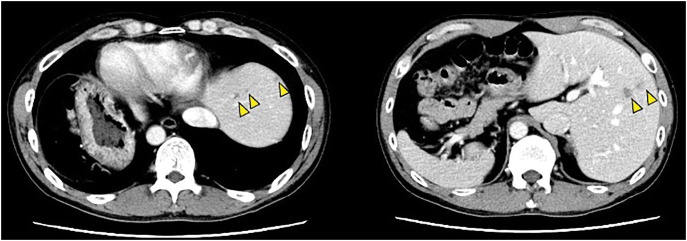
Contrast-enhanced CT showing multiple liver metastases (triangles).
